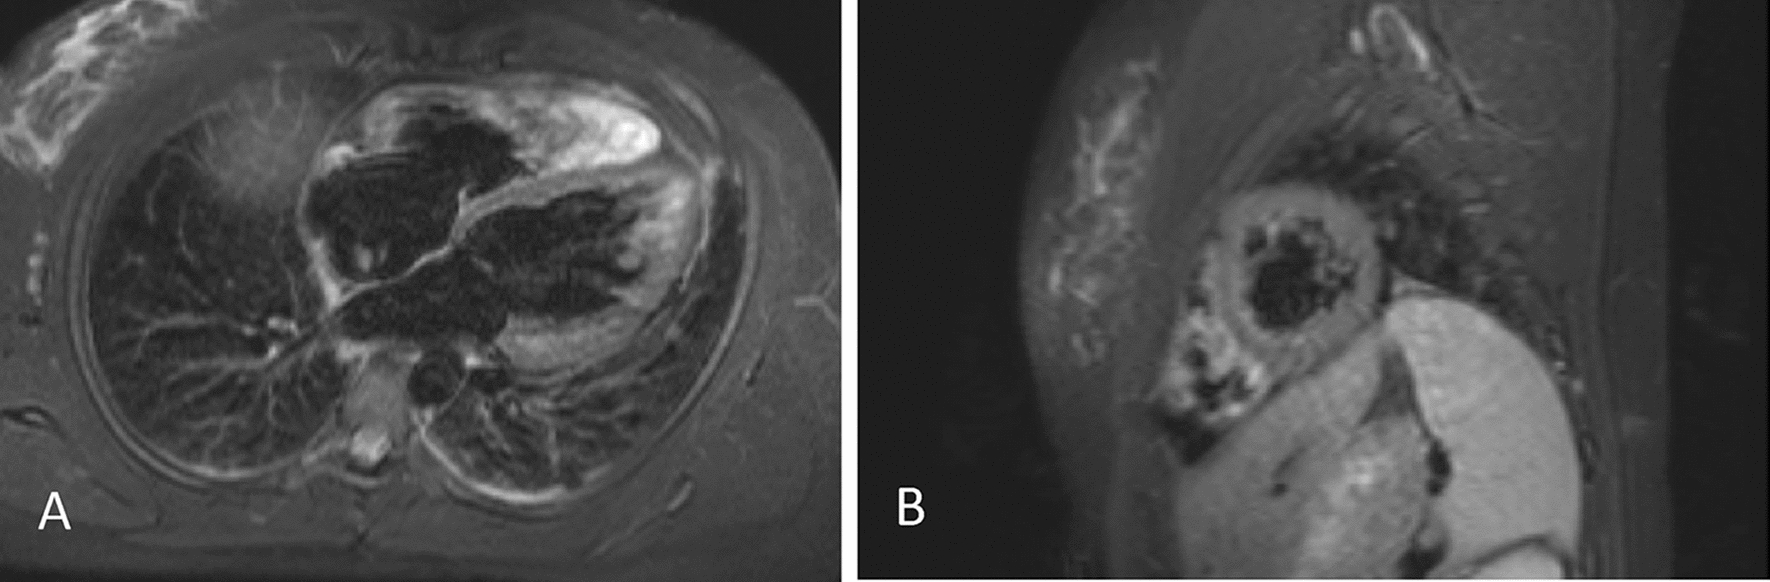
Evidence of Myocardial oedema on cardiac MRI post vaccination with BNT162b2. Transverse A and sagittal B, T2 STIR with gadolinium, images of cardiac MRI of Case 2 patient demonstrating myocardial oedema post vaccination with BNT162b2
